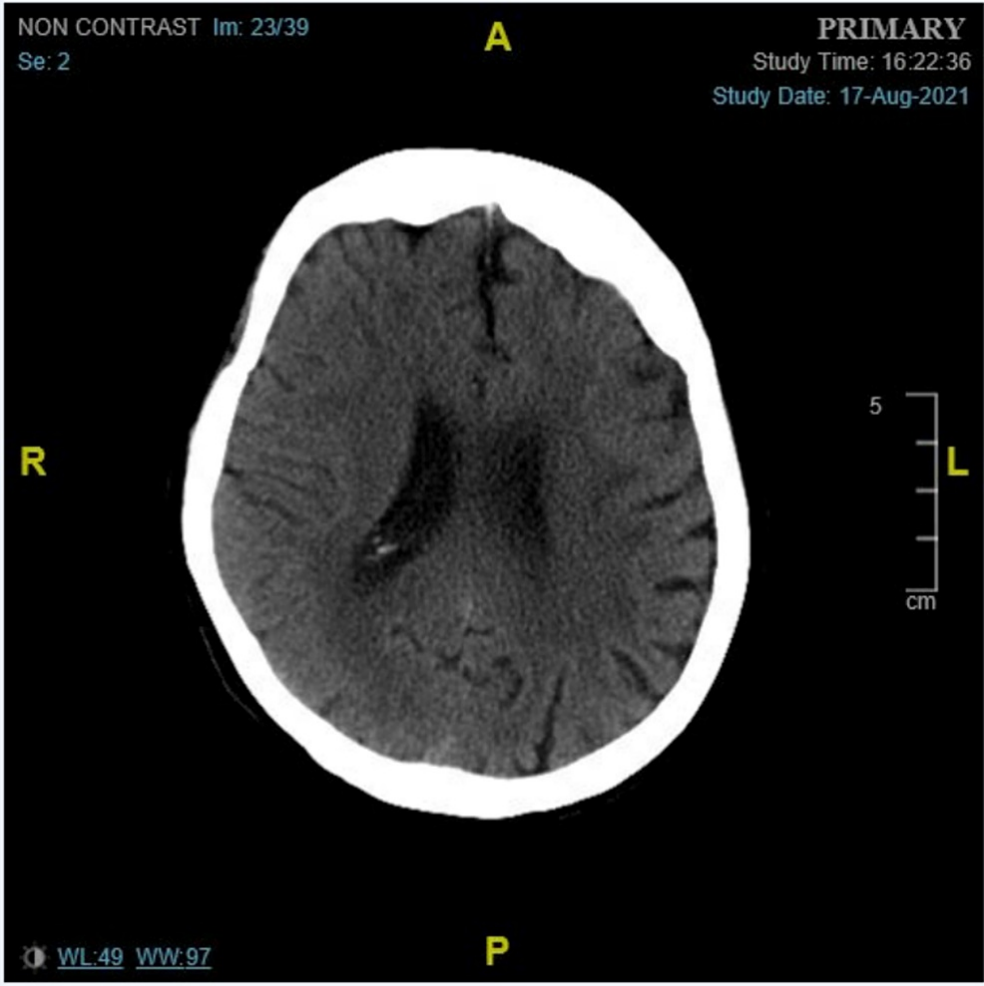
CT of the head showing areas of patchy low attenuation in the white matter of both cerebral hemispheres. CT: computed tomography
